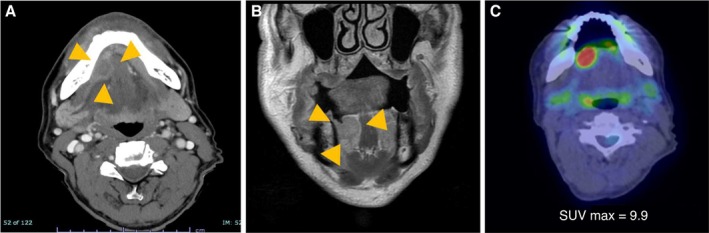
Radiological findings of the tumor. (A) Contrast‐enhanced computed tomography showed a 17 × 16‐mm enhancing mass in the right sublingual region, with no evidence of abnormal cervical lymphadenopathy or pulmonary lesion. (B) Contrast‐enhanced T1‐weighted magnetic resonance imaging showed the right sublingual gland contained a 21 × 15‐mm lesion with abnormal signal. (C) Fluorodeoxyglucose‐positron emission tomography/computed tomography showed intense uptake in the right sublingual region (standard uptake value [SUV] max 9.9) and no abnormal uptake elsewhere.
